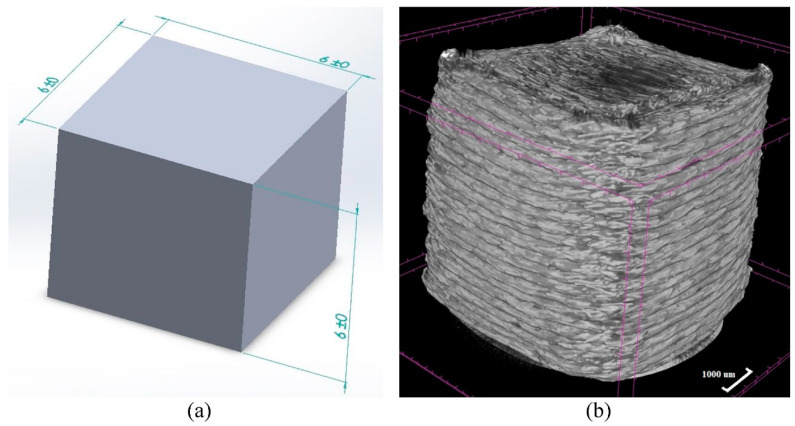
(a) CAD model with dimensions. (b) Micro-level CT scan of the 3D printed part [89] (reprinted with kind permission from Elsevier).
